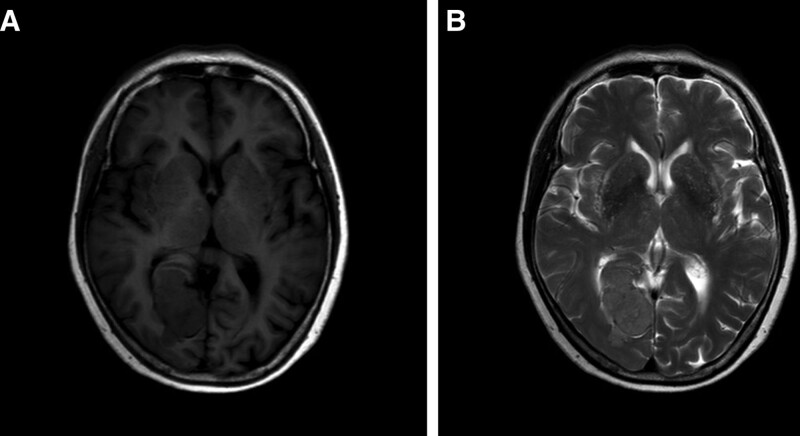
Cranial MRI in 2012: an oval like mass in the right occipital region with isointense on T1WI (A) and T2WI (B) without peritumoral edema, and maximum diameter about 3 cm. The posterior horn of the right ventricle is compressed. MRI = magnetic resonance imaging.
